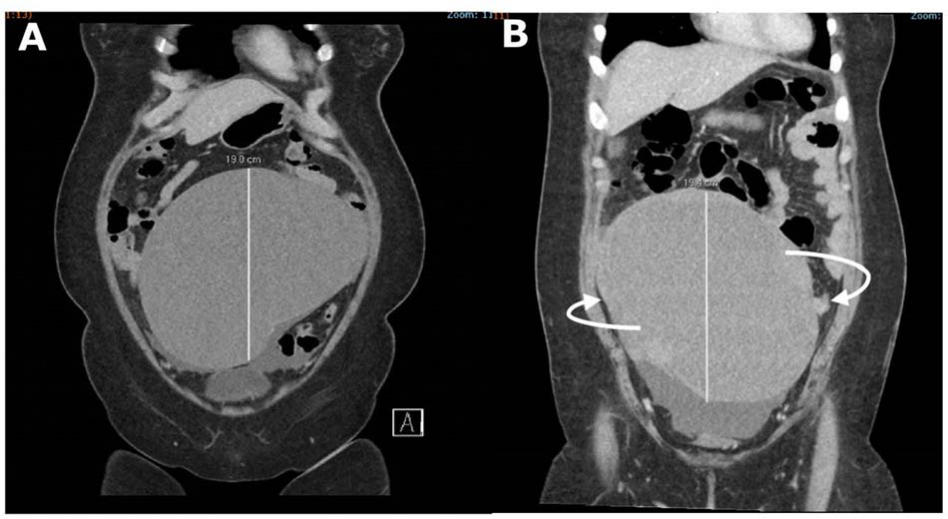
CT abdomen and pelvis (CTAP) showing acute change in orientation of the right ovarian mass before (A) and after (B) colonoscopy.
